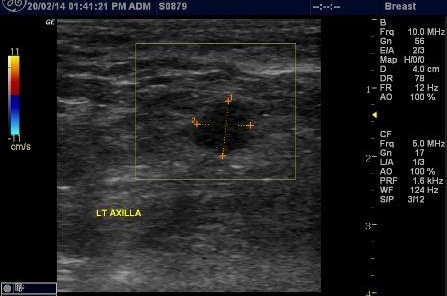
Ultrasound of the axilla with a replaced node showing an enlarged lymph node with irregular margins and loss of the usual corticomedullary differentiation; however, Doppler interrogation showed no increased vascularity.
